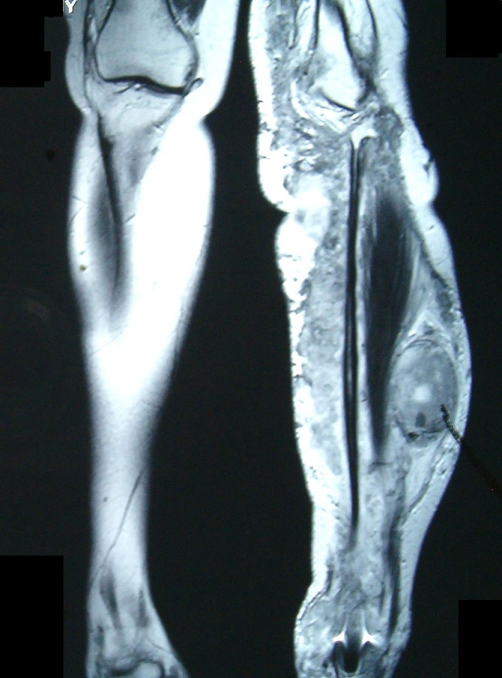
MRI image showing the site of the tumor in the left tibia (Case 1).
